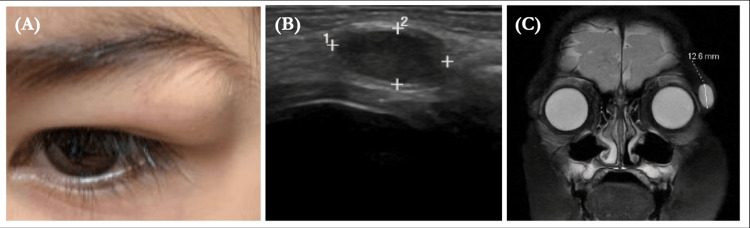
Pediatric patient with a well-defined mass on his left upper eyelid. Panel A shows the gross appearance of the mass at the patient’s initial ophthalmology appointment, panel B the ultrasound, and panel C the computed tomography (CT) scan. All images in this panel are original.
